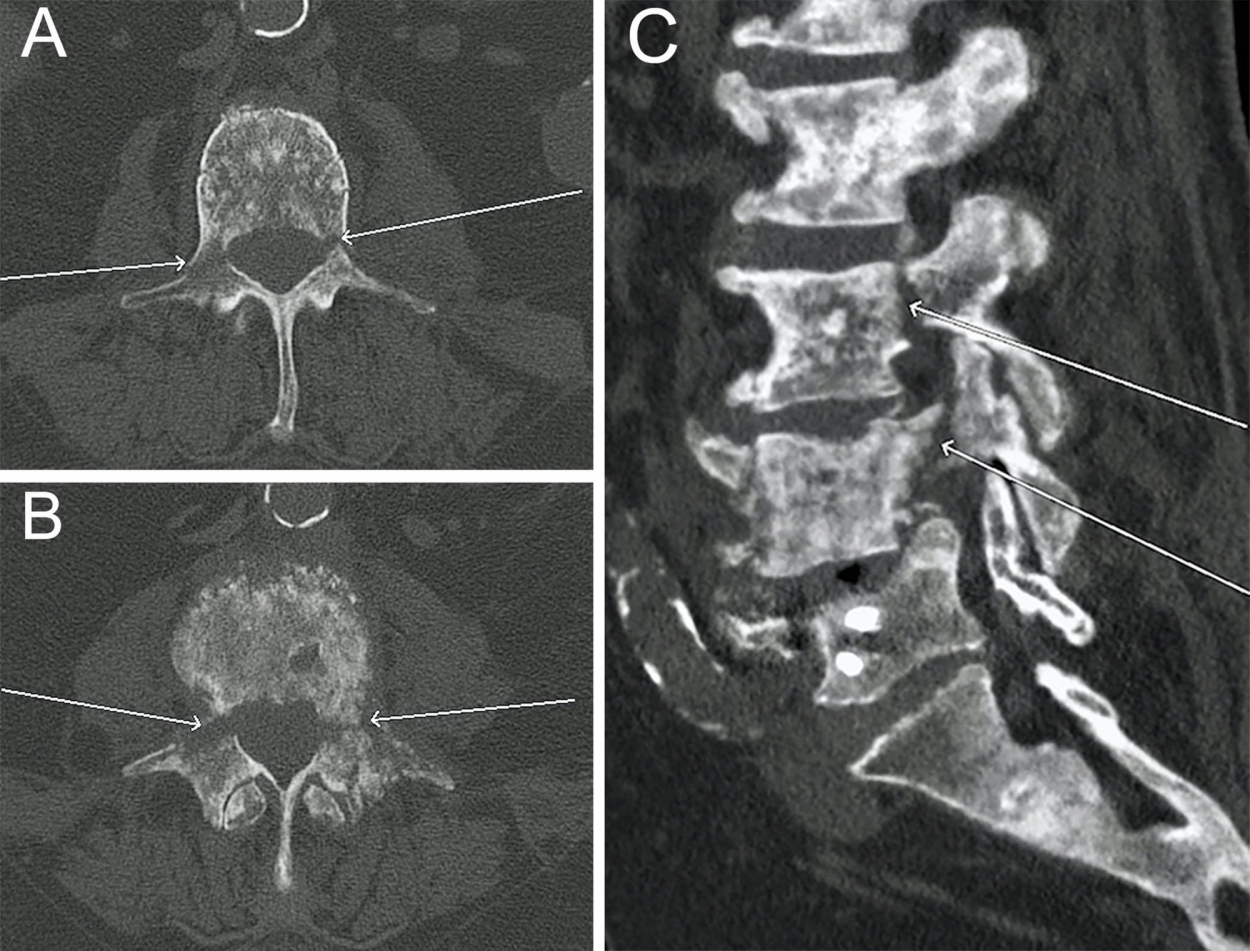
Preoperative computed tomography (CT) images of the lumbar spine (A) and (B): Axial computed tomography (CT) imaging of the lumbar spine demonstrating bilateral pedicle fractures at the third and fourth lumbar levels (L3 and L4, respectively) with pathologic compression fracture at L4. White arrows in both figures point to pedicle fracture lines. (C) Sagittal plane CT revealing pedicle fractures at L3 and L4 (white arrows) and tumor infiltration of the L3 and L4 vertebral bodies.
